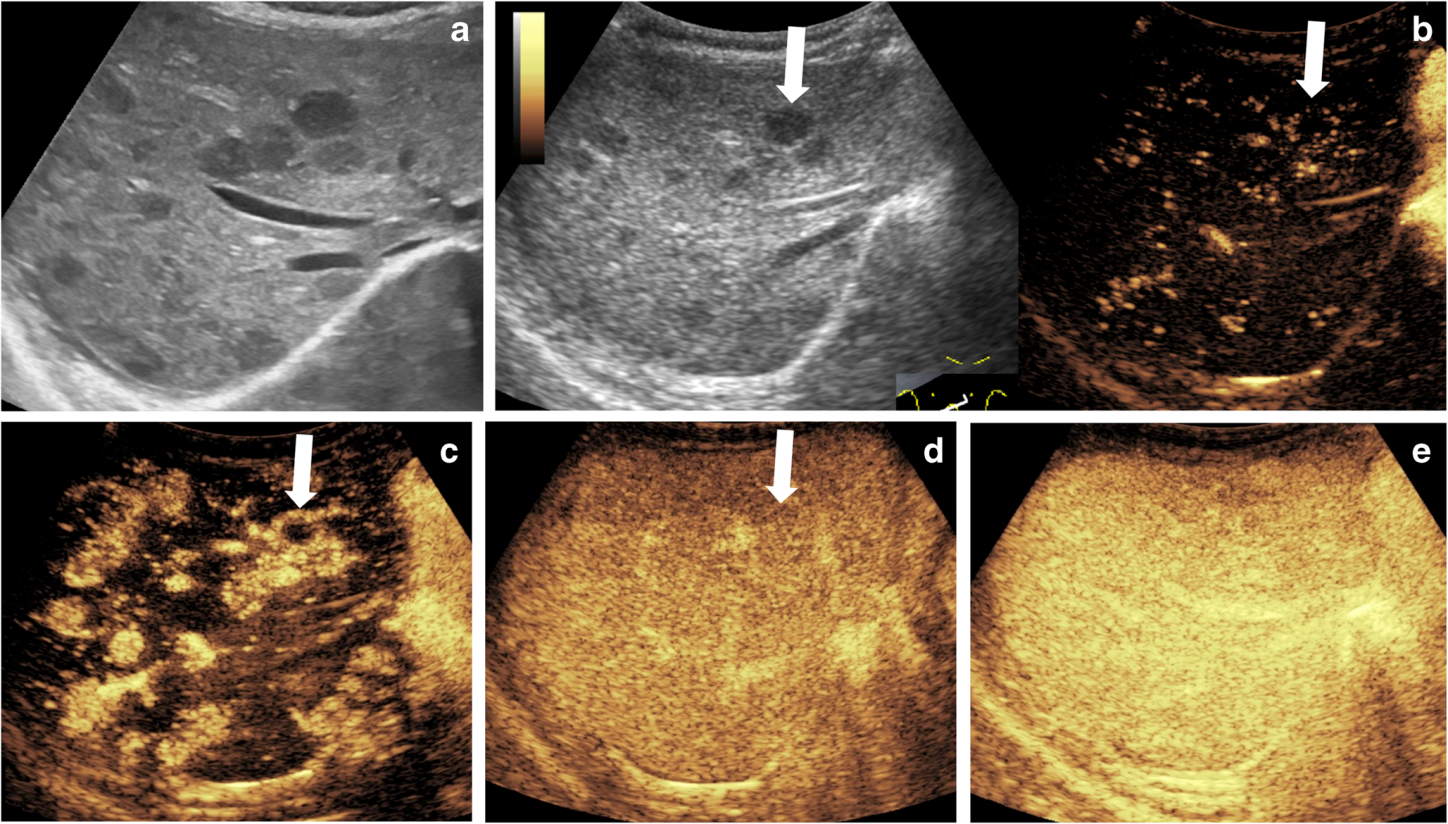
Incidental liver hemangiomatosis in a 4-month-old boy. a Transverse gray-scale US shows multiple hypoechoic lesions throughout the liver. b–e Transverse contrast-enhanced ultrasound (CEUS) of the liver. Dual display of the gray-scale (*left*) and contrast-enhanced (*right*) image at 8 s (b) and contrast-only CEUS images at 10 s (c), 22 s (d) and 1 min 33 s (e) after contrast administration. Early peripheral enhancement is exemplified in the largest lesion (*arrows*), followed by centripetal enhancement and nearly complete and homogeneous enhancement of all lesions similar to the background hepatic parenchyma. No washout is noted in any of the lesions (e)
